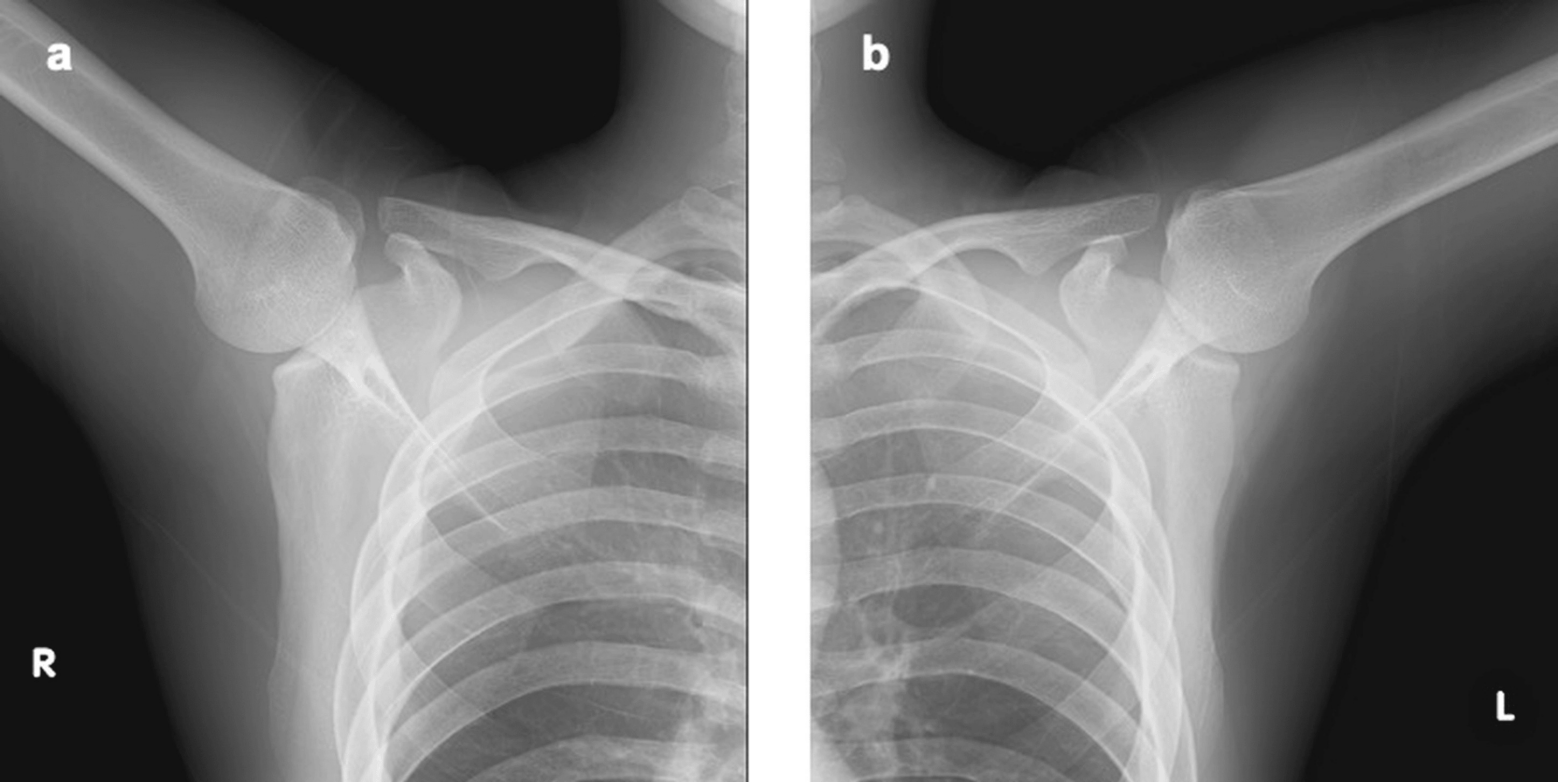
Plain radiographs of the shoulder. Upright radiographs obtained with the arm in an elevated-arm position showed no abnormalities. a) right shoulder, b) left shoulder.
